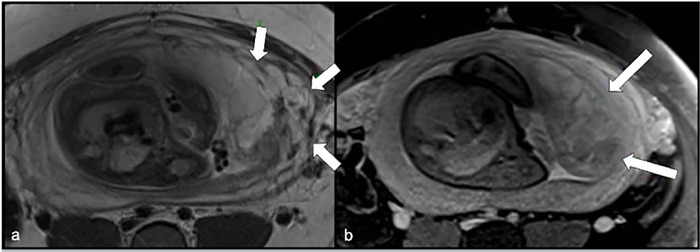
Axial (a) T2-weighted and (b) T1-weighted images. Areas of an undefined
